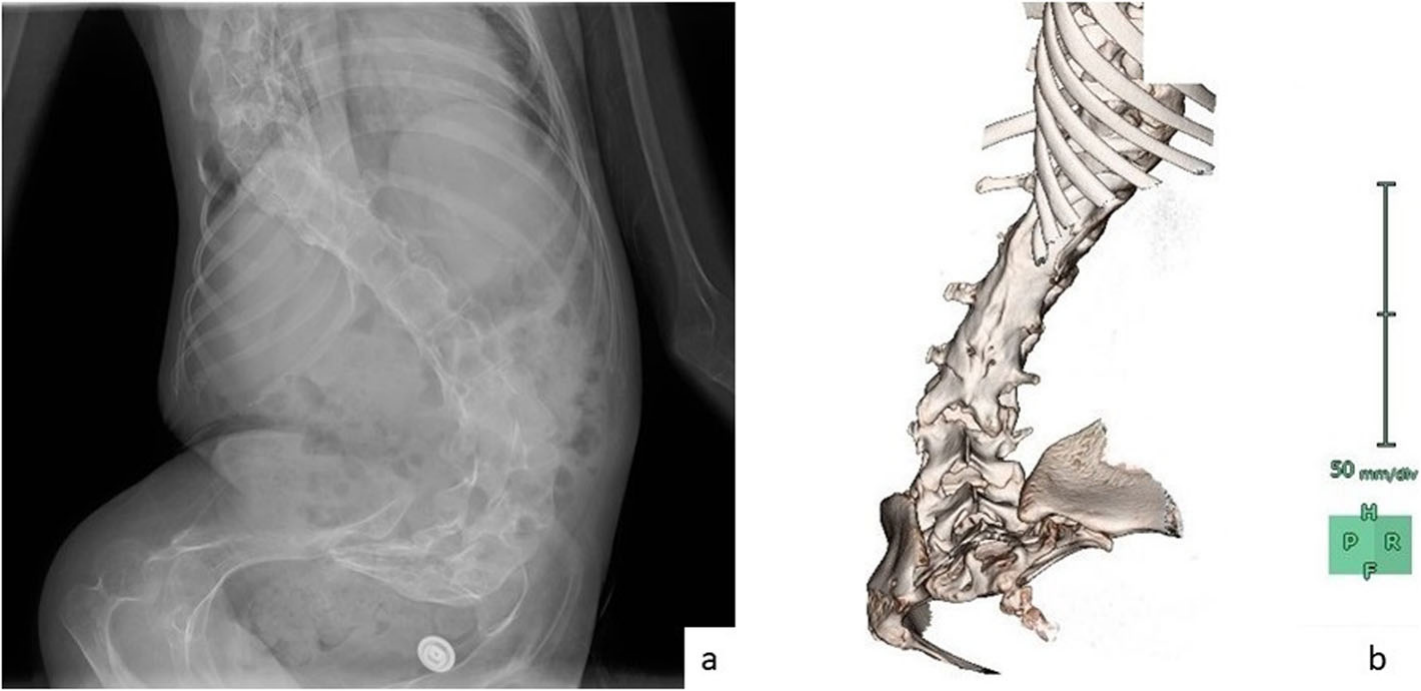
a A plain anteroposterior X-ray image of the vertebrae. b A three-dimensional reconstructed image of CT scan. A view from the back shows rotation of the vertebrae and fused posterior segment
